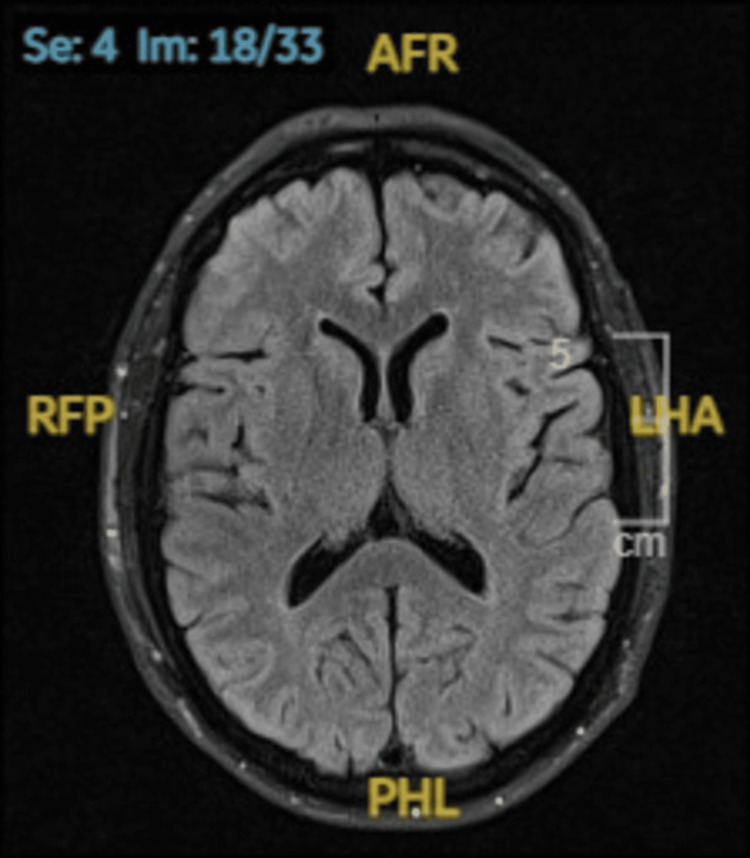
Axial brain FLAIR MRI sequence demonstrating normal cerebral structures with no evidence of acute infarction, haemorrhage, or mass lesion Image exported from the hospital radiology PACS and anonymised for publication FLAIR: fluid-attenuated inversion recovery, MRI: magnetic resonance imaging, PACS: Picture Archiving and Communication System
